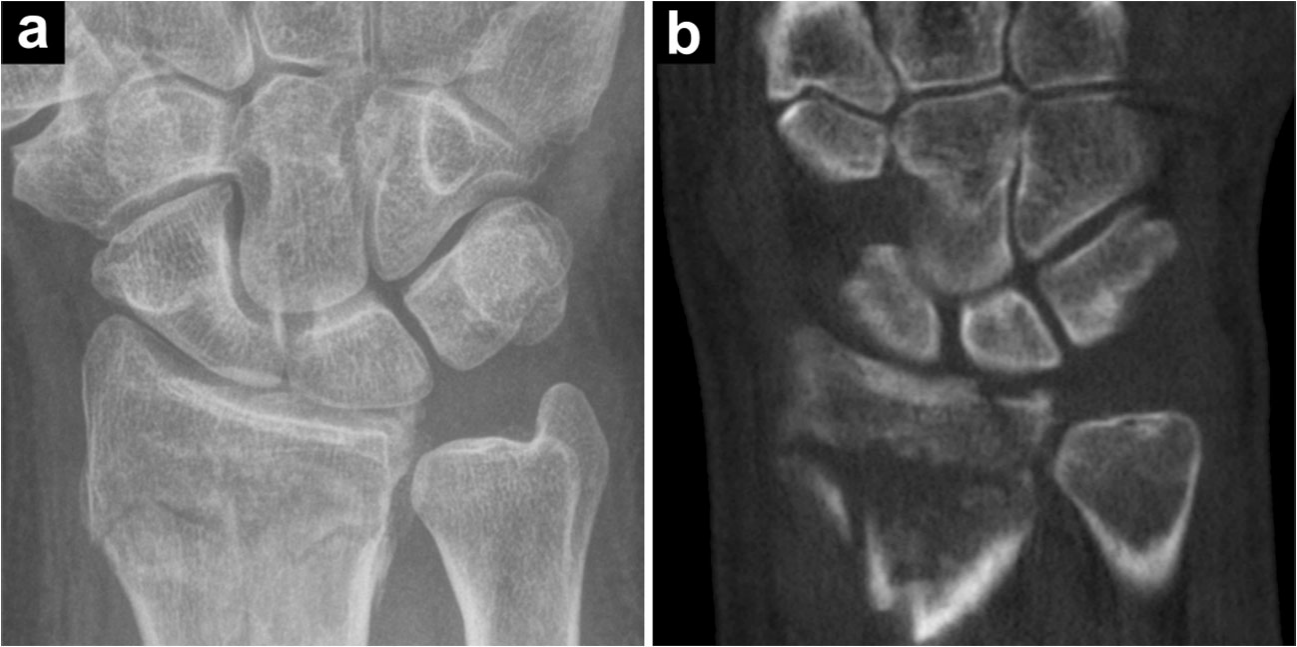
After a fall on the outstretched hand, x-rays of a 63-year-old man exhibited a distal radius fracture with metaphyseal impaction (a). While articular surface affliction could not be ascertained in radiography, the cone-beam CT scan demonstrated involvement of the radiocarpal and distal radioulnar joint (b). Open reduction was advised irrespective of CT imaging and the reported impaction of the lunate fossa was corrected intraoperatively
